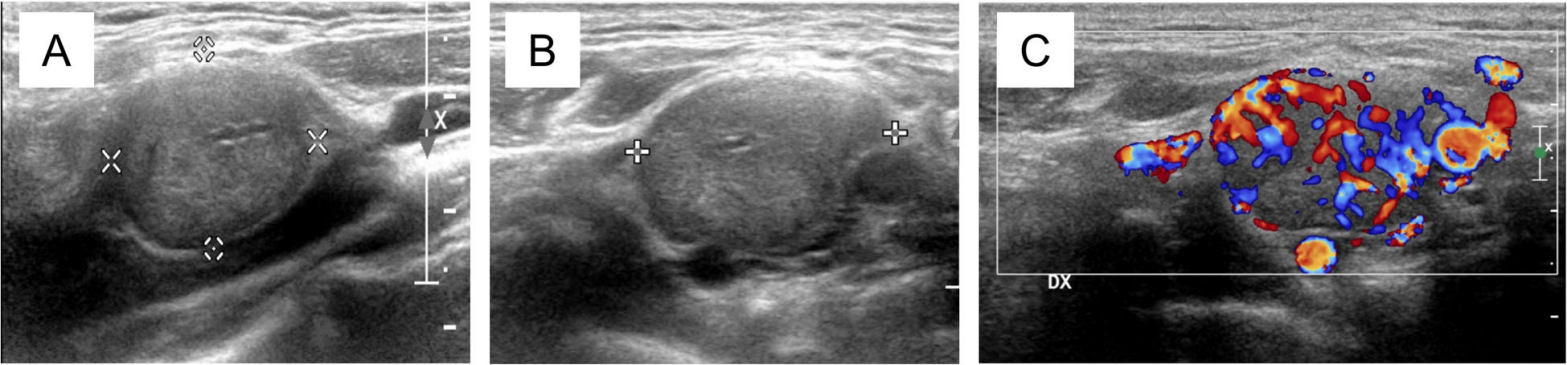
Neck ultrasonography (a) and b ultrasonography B-mode paraganglioma images; c axial color-Doppler ultrasonography image
